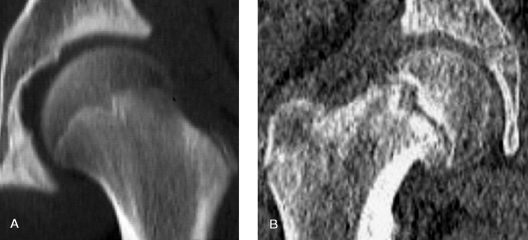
A. An AP CT scanogram of the left hip of a 14-year-old boy showing a well-developed epiphyseal peg. B. A similar CT scanogram of the right hip of a 13-year-old boy who has a poorly defined peg that appears to be showing signs of loosening.
